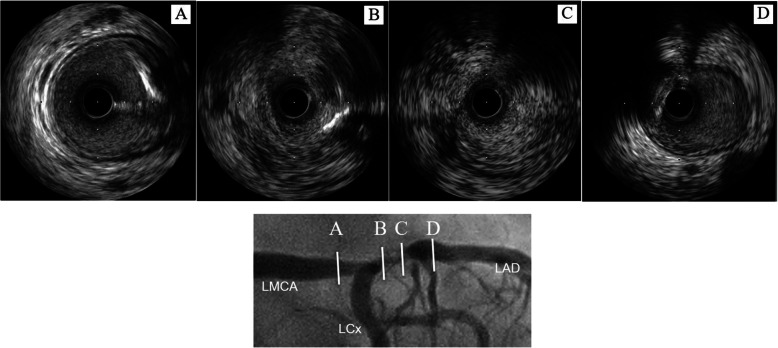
Intravascular ultrasound images. Intravascular ultrasound images of the stenotic lesion obtained during the first coronary intervention showed a fibrotic intimal thickness and an attenuated plaque was not seen. It is noteworthy that the media was partly unrecognizable and the echo intensity similar to fibrotic intimal thickening traversed from the intima to the adventitia, thereby causing the whole image of the coronary artery to become unclear
